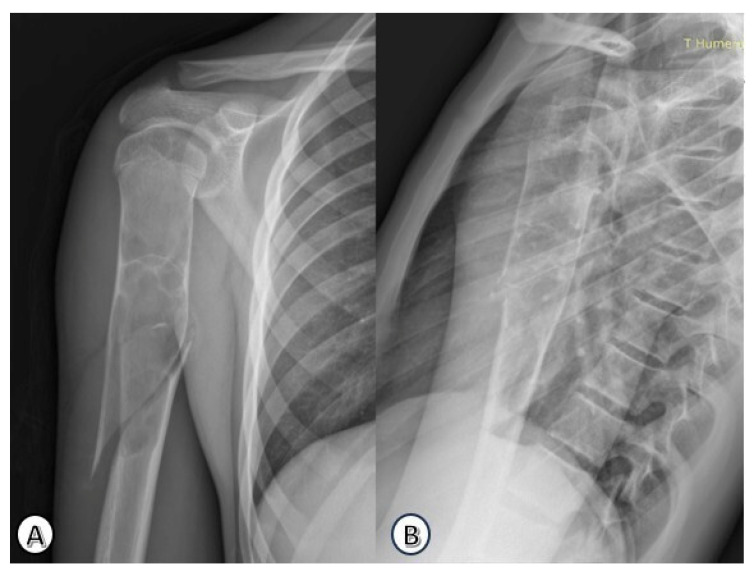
Preoperative anteroposterior (A) and lateral (B) radiographs of the right humerus revealing a simple bone cyst with an associated pathological spiral fracture in a 16-year-old male patient.
